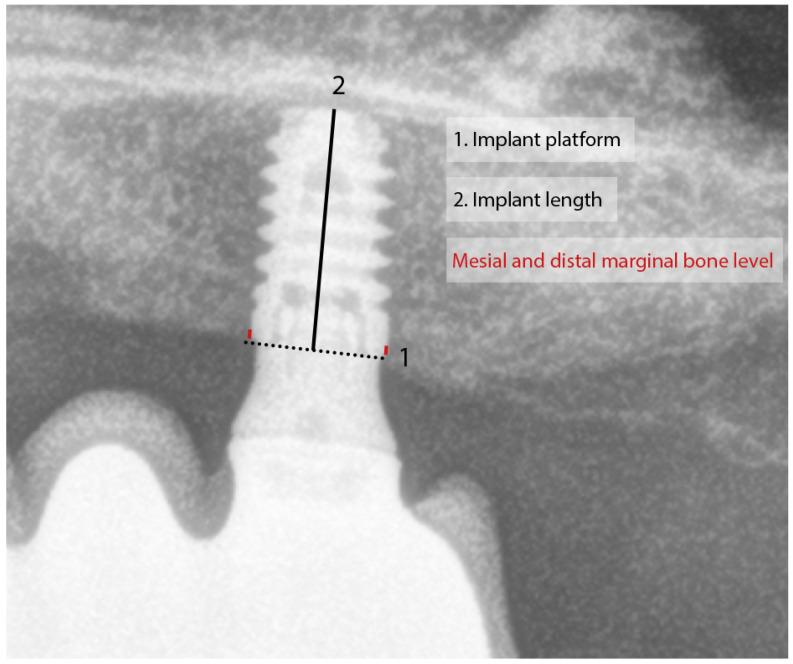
Measurement of the radiographic marginal bone level. The known length of the implant was used for measurement calibration. Mesial and distal marginal bone level was measured from the implant platform to the most coronal point of implant–bone contact.
